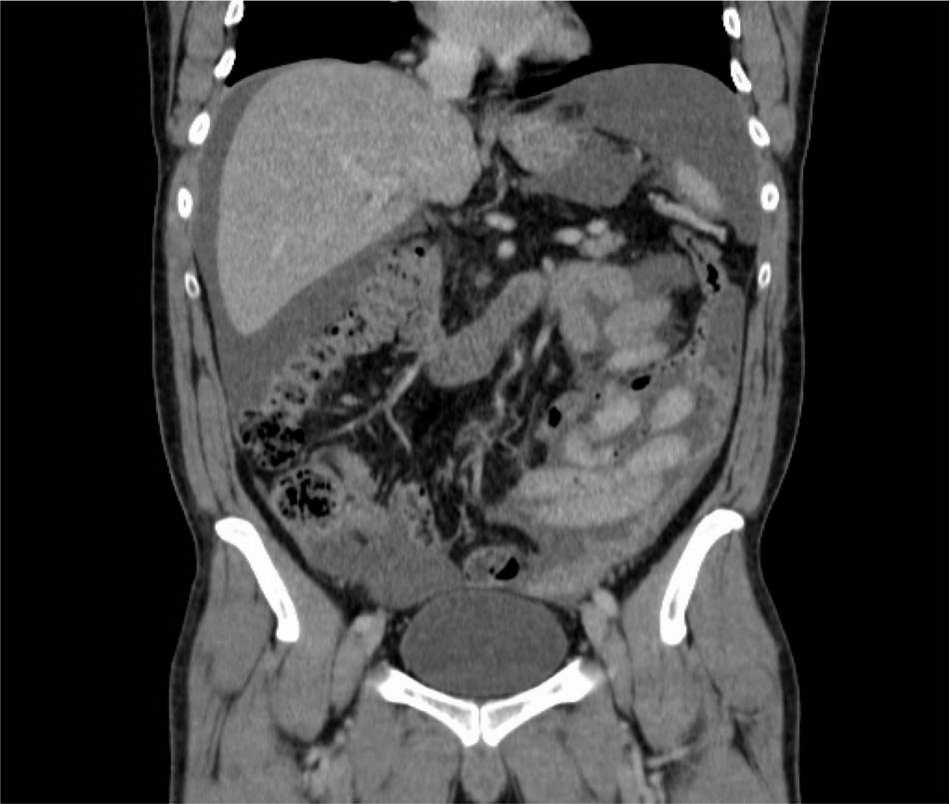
Increase in the amount of ascites post-ultrasound-guided biopsy.
